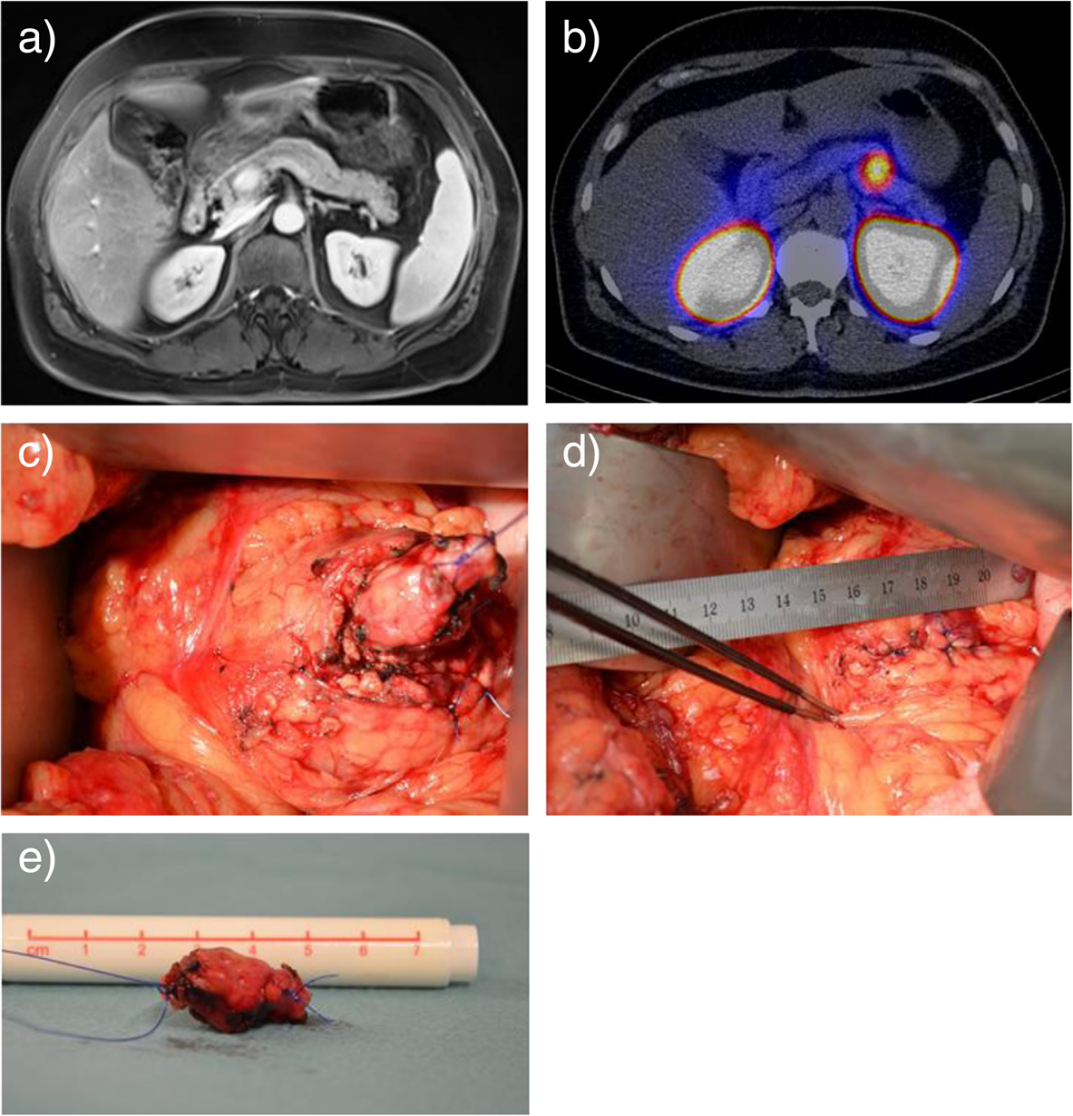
a) and b) MRI without pathological finding in the pancreatic corpus whereas ^111^In-DOTA-exndin-4 SPECT/CT detects the insulinoma. c) Enucleation was performed and d) the pancreatic capsule was closed by direct suture. e) The insulinoma (14 mm) was surrounded by a minimal mass of healthy tissue.
